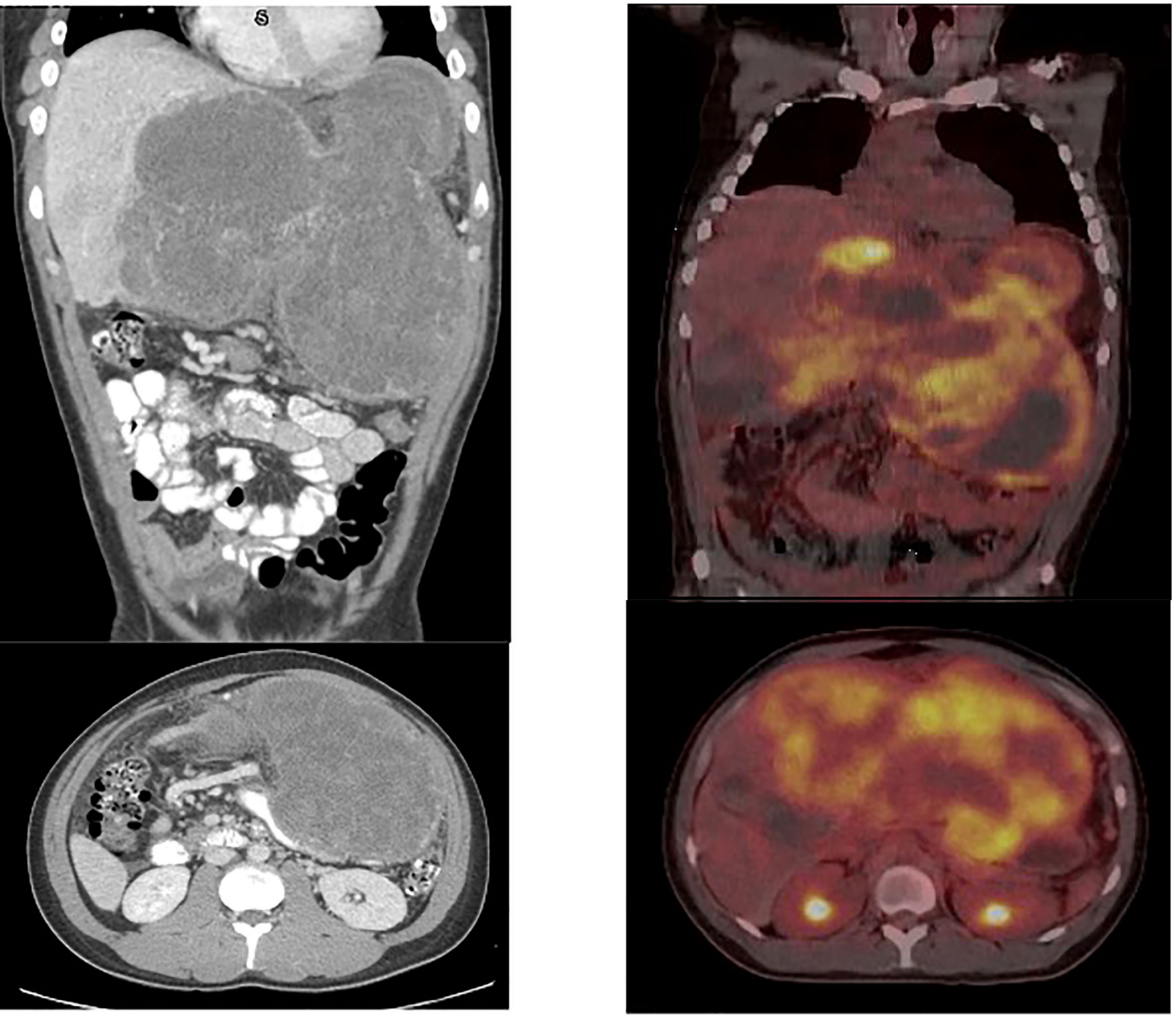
Initial CT scan demonstrating UESL. 20 x 10 x 10 cm well-circumscribed, solid mass of the entirety of the left lobe of the liver with relative sparing of the right lobe. Initial PET/CT demonstrating large hepatic mass measuring 25 x 13 cm with maximal SUV 11.5 as well as areas of necrosis, demonstrated by lack of FDG avidity.
